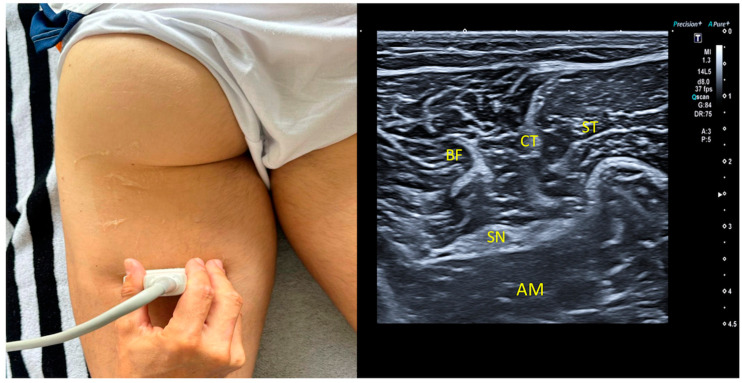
SN: sciatic nerve; CT: conjoint tendon; BF: biceps femoris; ST: semitendinous; SMT: semimembranosus tendon; AM: adductor magnus.
